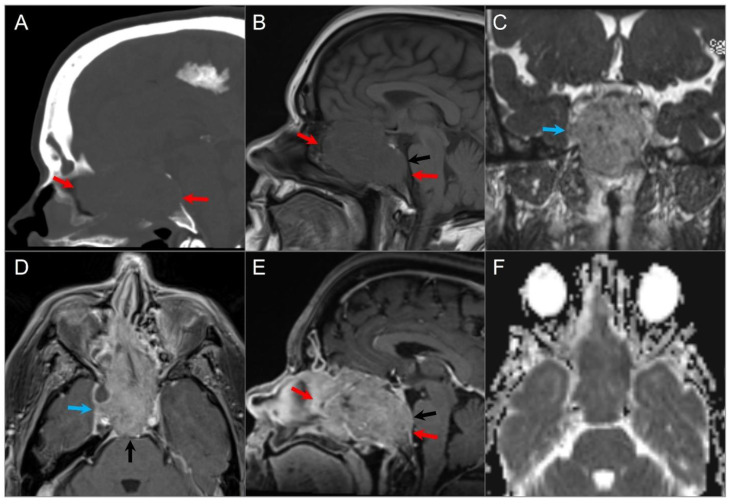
Skull base invasion of squamous cell Ca. (A) Sagittal CT image shows a hypodense soft tissue mass lesion centered in the superior nasal cavity, ethmoid, and sphenoid sinuses, which erodes the anterior skull base, sellar floor, and clivus (red arrows). (B) Sagittal noncontrast T1W, (C) coronal postcontrast CISS, (D) axial and (E) sagittal postcontrast T1W images demonstrate significant heterogeneous enhancement of the lesion with extension into the right cavernous sinus (blue arrow), prepontine cistern (black arrows), and anterior cranial fossa. (F) ADC map image shows mild restricted diffusion in the lesion, suggesting high cellularity.
